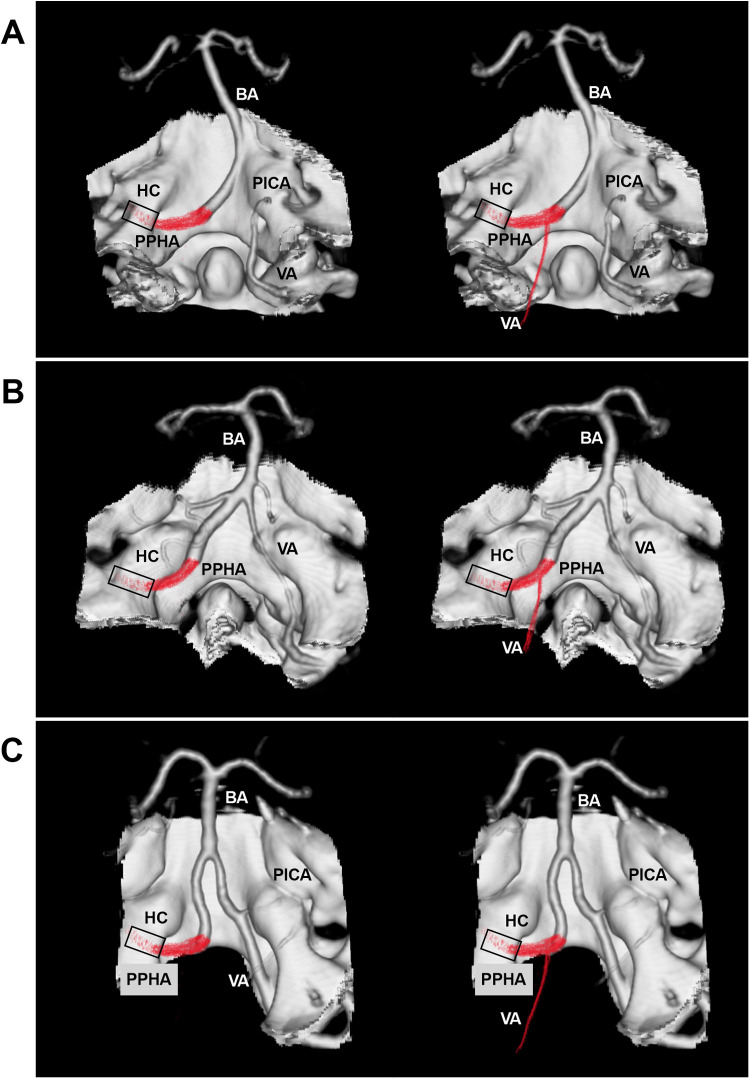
Relationship between the PPHA and VA on hybrid CTA. In all panels, hybrid CTA images show the PPHA passing through the HC to replace the VA. (A) The contralateral VA is hypoplastic and terminates in the PICA; the ipsilateral VA is absent (left panel) or hypoplastic (right panel). (B) The contralateral hypoplastic VA connects to the BA; the ipsilateral VA is absent (left panel) or hypoplastic (right panel). (C) The contralateral VA is normal; the ipsilateral VA is absent (left panel) or hypoplastic (right panel). BA, basilar artery; CTA, computed tomography angiography; HC, hypoglossal canal; PICA, posterior inferior cerebellar artery; PPHA, persistent primitive hypoglossal artery; VA, vertebral artery.
